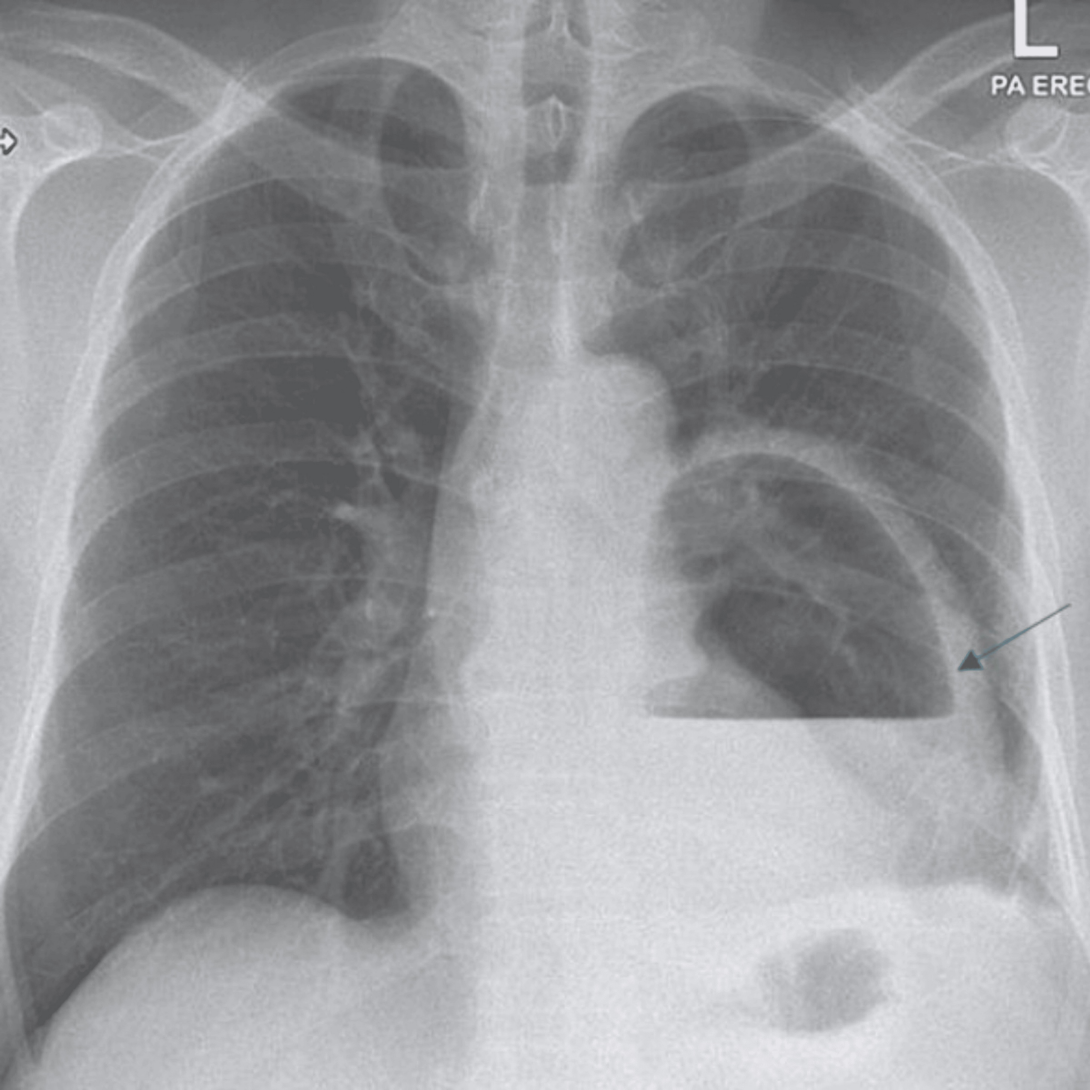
Chest X-ray. Posteroanterior chest radiograph demonstrating a large cavitary lesion in the left lower lung zone. A prominent air-fluid level (arrow) is visible within the cavity, a characteristic finding of a lung abscess.
